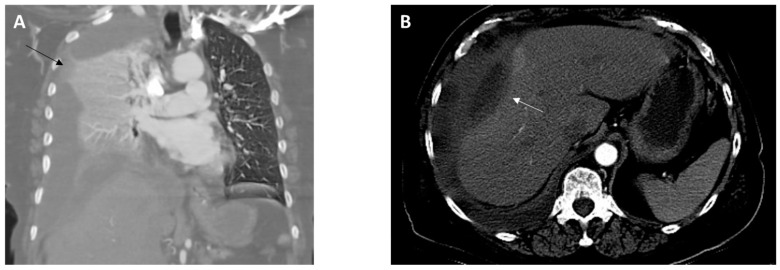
Images from computed tomography angiography of the chest obtained at admission. (A), Coronal view revealing a collapsed right lung due to a large pleural effusion (black arrow). (B), Axial view showing the presence of a perihepatic abscess (10.0 × 3.8 cm) (white arrow).
